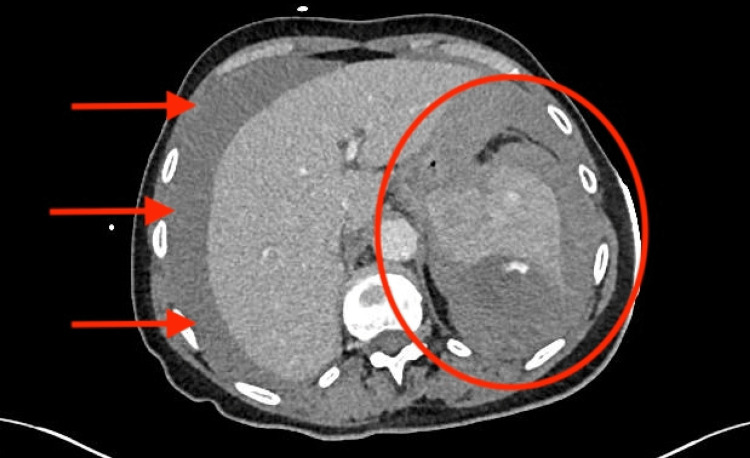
Axial CT abdomen/pelvis at a more inferior splenic level demonstrating the further extent of hemoperitoneum and dependent fluid collection. The red arrow highlights the contrast extravasation, and the red circle indicates the main region/site of hemorrhage.
